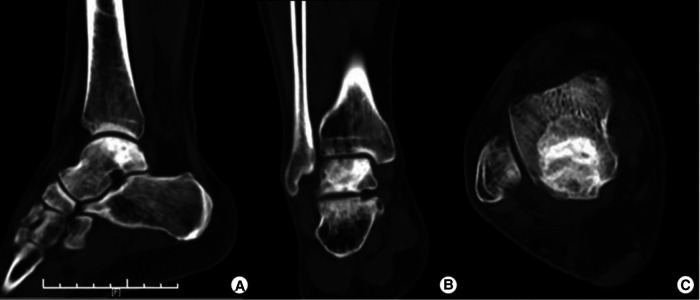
One-year postoperative CT images showing good bone remodeling and osteointegration with no tumor recurrence.
